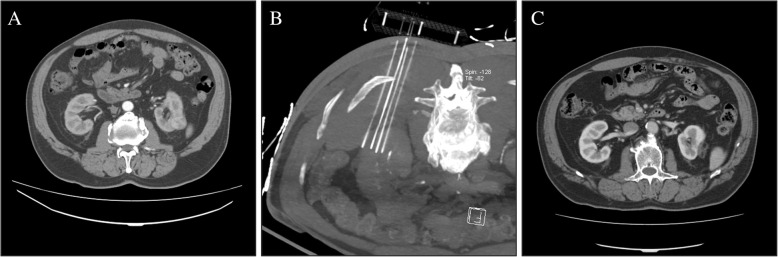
a Contrast-enhanced CT transversal image before IRE with the tumor located in the middle pole of the left kidney. b Reconstructions of the CT scan after the placement of four needles. c Contrast-enhanced CT axial image 18 months after IRE. The renal mass showed no contrast enhancement. The maximal diameter of the tumor was reduced compared with that in the baseline image
